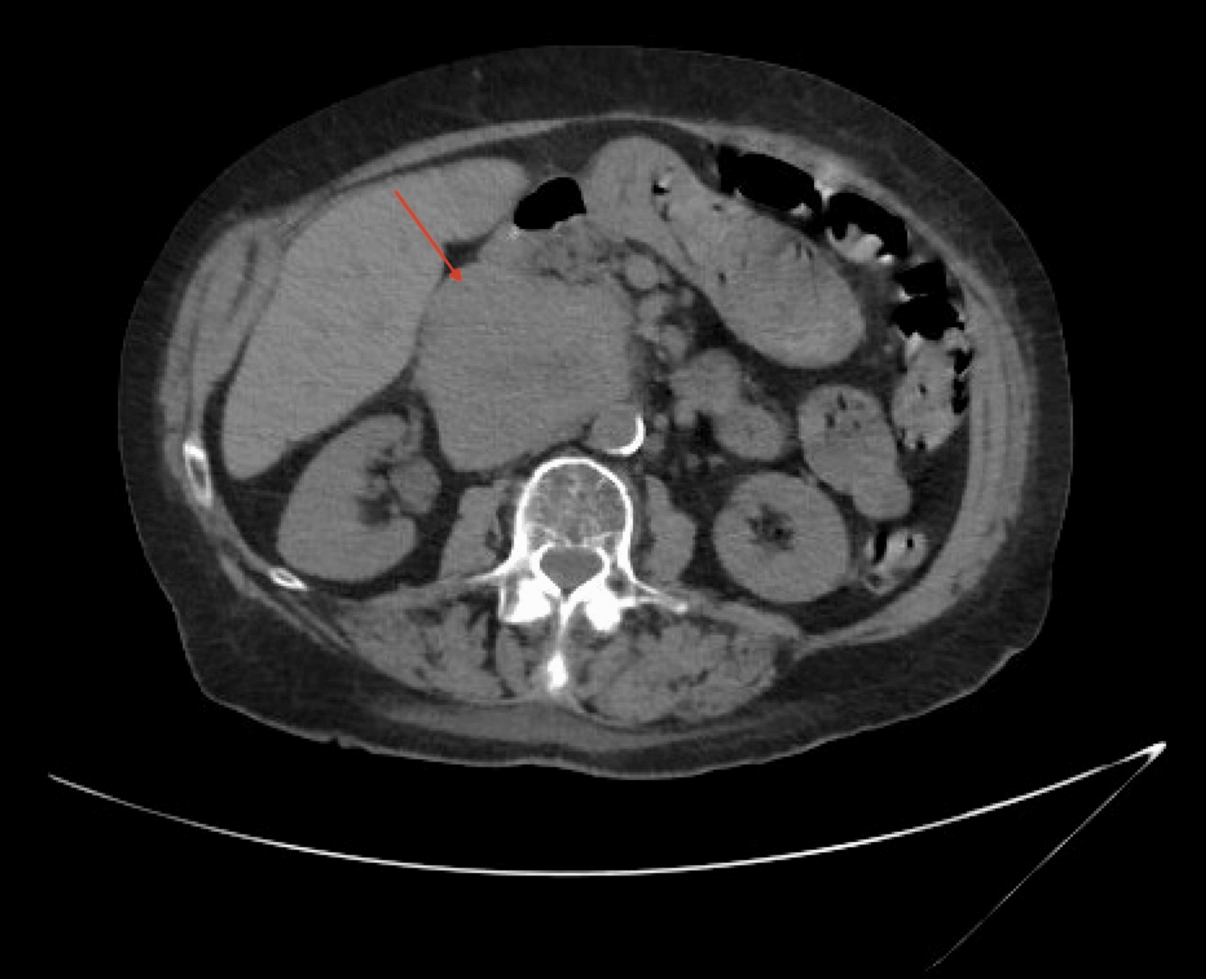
CT of the abdomen/pelvis with contrast showing a large retroperitoneal mass compressing the inferior vena cava.
